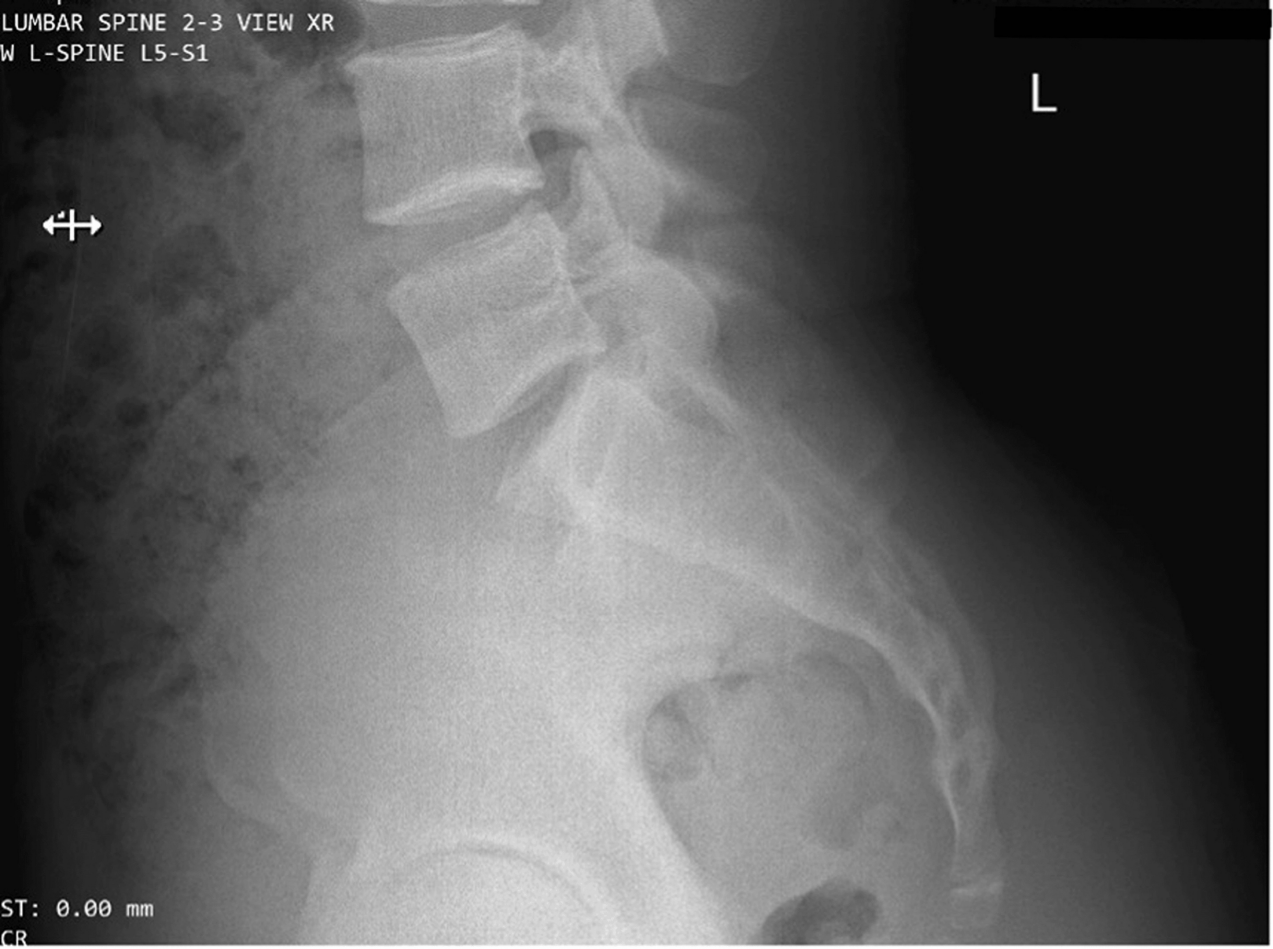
Lumbosacral spine lateral view plain films read as unremarkable for this patient, with the fifth lumbar-first sacral (L5-S1) conjoined nerve roots not readily identifiable.
